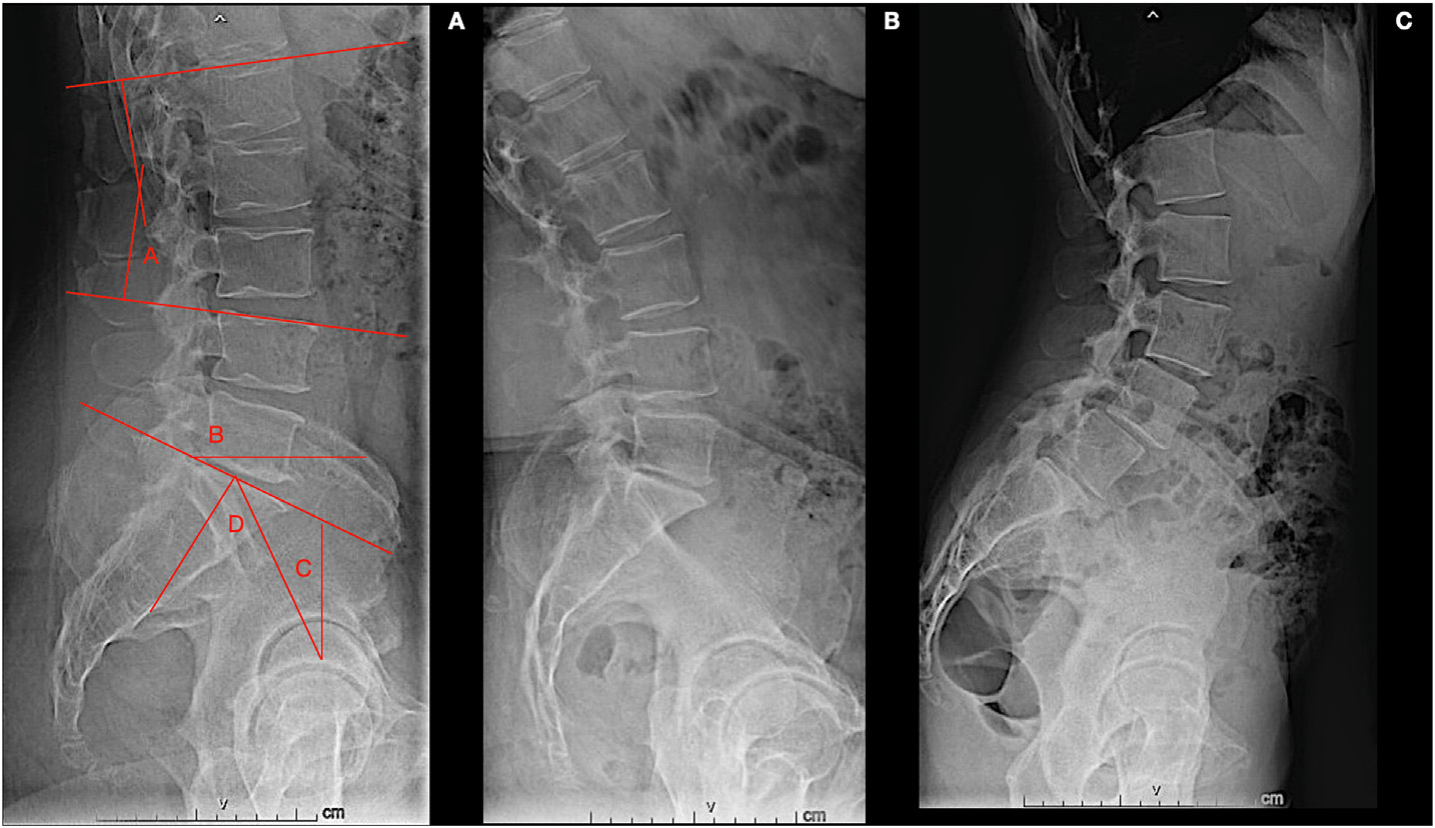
The figure shows the main Sagittal balance parameters used for the study (part A) namely Lumbar lordosis (red A), Sacral slope (red B), Pelvic Tilt (red C), Pelvic incidence (red D) and as can be seen in the X-ray images in maximum extension (part B) and maximum flexion (part C) of the patients, they can vary even significantly.
